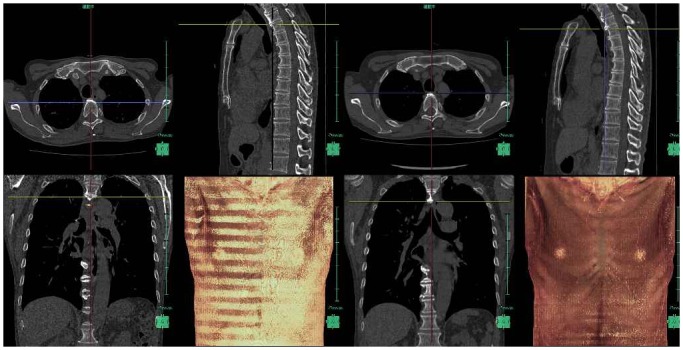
Original temporal 3D-CT subtraction CAD on the screen. The left half of the screen displays the follow-up CT images, and the right half of the screen displays the initial CT images.
